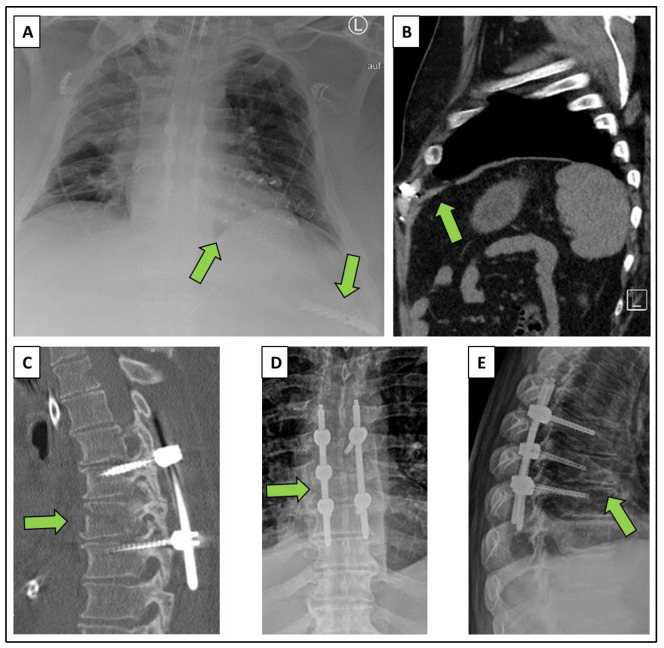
Postoperative X-ray and CT scan: (A) thoracic anterior-posterior view, (B,C) sagittal CT slices, (D) anterior-posterior view of the spine, (E) lateral view of the spine. The respective injuries and their treatment are marked with arrows. A multistage procedure with a focus on damage control surgery was performed first. The severe rupture of the diaphragm had been fixed in a supine position (Figure 2B). Once the patient had been stabilized via intensive care treatment, the next step on the following day was the fixation of the thoracic vertebral spine in a prone position by dorsal instrumentation with an internal screw rod system (Viper, DePuySynthes, Zuchwil, Switzerland) resulting in anatomic spinal alignment (Figure 2C). Operative treatment had been completed by second look thoracotomy after three days in a lateral decubitus position. Within this procedure, surgical stabilization of rib fractures (SSRF; plates and locked screws) had been included as well as the closure of the intercostal hernia by direct suture of the intercostal muscle layers (Figure 2A). The lower costal margin has been fixed with a locking plate at the confluence of the 7th and the 8th rib. Postoperative X-ray of the chest (Figure 2A) and postoperative CT scan (Figure 2B) show normal intercostal spaces and normal shape of the diaphragm. The patient was able to receive follow-up rehabilitation after discharge and was back to work without any restriction in neurological outcome (5/5 points on Glasgow Outcome Scale). The combined thoracovertebral injury entity seems to be rare and has hardly been researched yet. Injuries with the so-called hyperextension-distraction mechanism of the human trunk are considered to be particularly risky. Individual cases with a ruptured spinal fracture and an anterior rupture of the chest wall with torn sternum are rarely described, probably because most patients already die at the accident scene [1]. A similar accident mechanism can analogously lead to a rupture of the thoracic wall and a simultaneous spinal column fracture [1,2,3]. This case indicates that hyperextension fractures of the thoracic spine may result in high injury severity and overall life-threatening thoracoabdominal injuries once the lower ribs at the costal margin show a fracture at the same time. The energy apparently continued through the thoracoabdominal organs over the intercostal space to the costal arch. This created an unstable situation for the anterior and lateral chest wall. We see the restoration of the stability as a supporting argument for our surgical treatment strategy of the thorax in case of this severe combined injury entity. Equivalently, operative stabilization was recommended for similar injury patterns [4,5,6]. The peculiarity of the injury combination of a distraction injury to the thoracic spine plus a fracture of the costal arch is above all the risk of two major accompanying injuries: an intercostal tear with consecutive lung herniation and a diaphragmatic tear leading to a consecutive two-cavity injury. The indicator function for a severe trauma is again underlined: despite the severity of the associated injuries, two-cavity traumata and particularly diaphragmatic injuries are often diagnosed only secondarily and delayed [4,5]. Because of their association with high overall injury severity, injuries to the costal arch should be detected or excluded by thorough physical examination and assessment of the trauma CT in the soft tissue window. This is rather unique in chest wall trauma and makes a difference to serial rib fractures in their shaft segment. Since we assume an underestimation of this combined injury entity, this clinical recommendation could lead to improved detection of potential associated life-threatening accompanying thoracoabdominal injuries.
